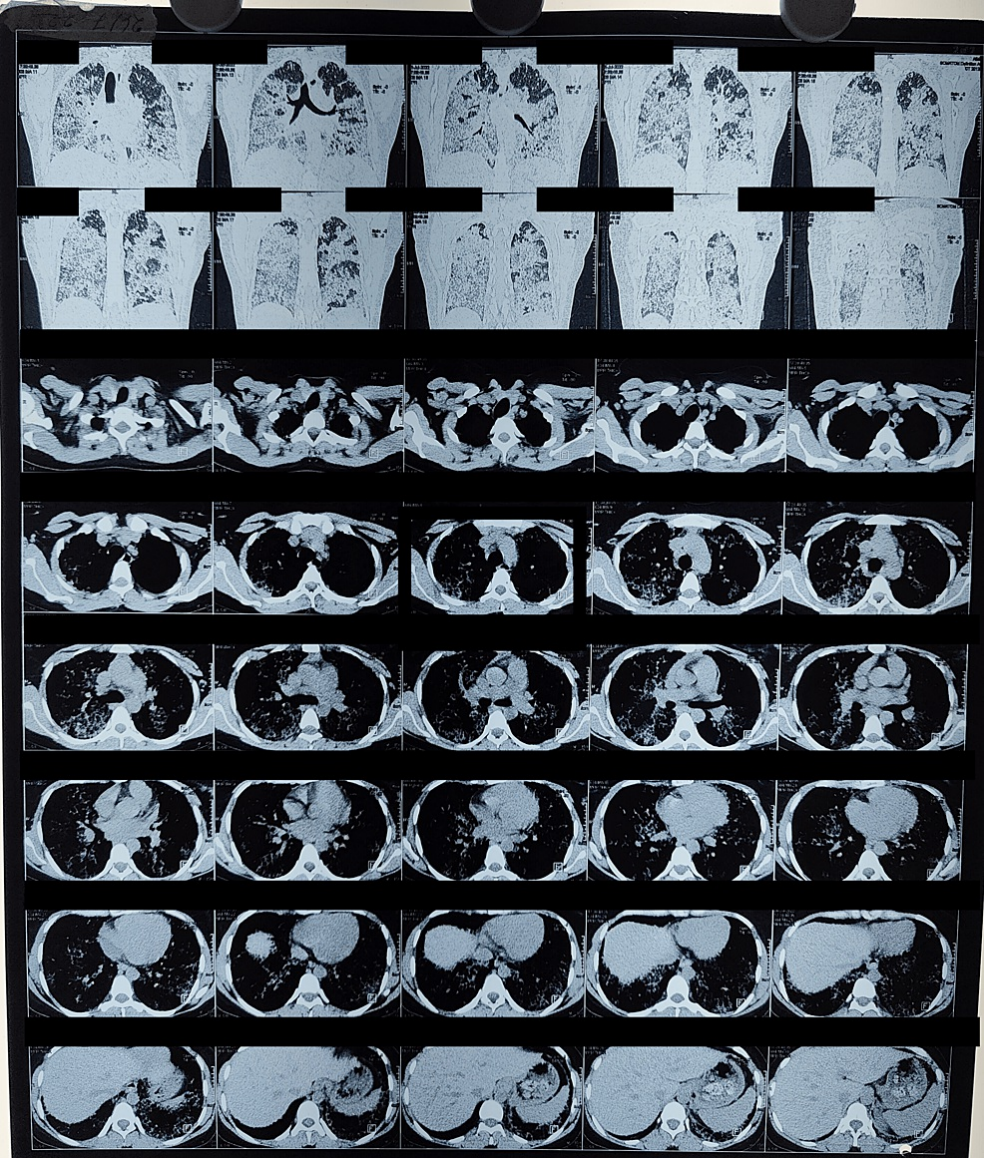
HRCT thorax HRCT: high-resolution computerized tomography
